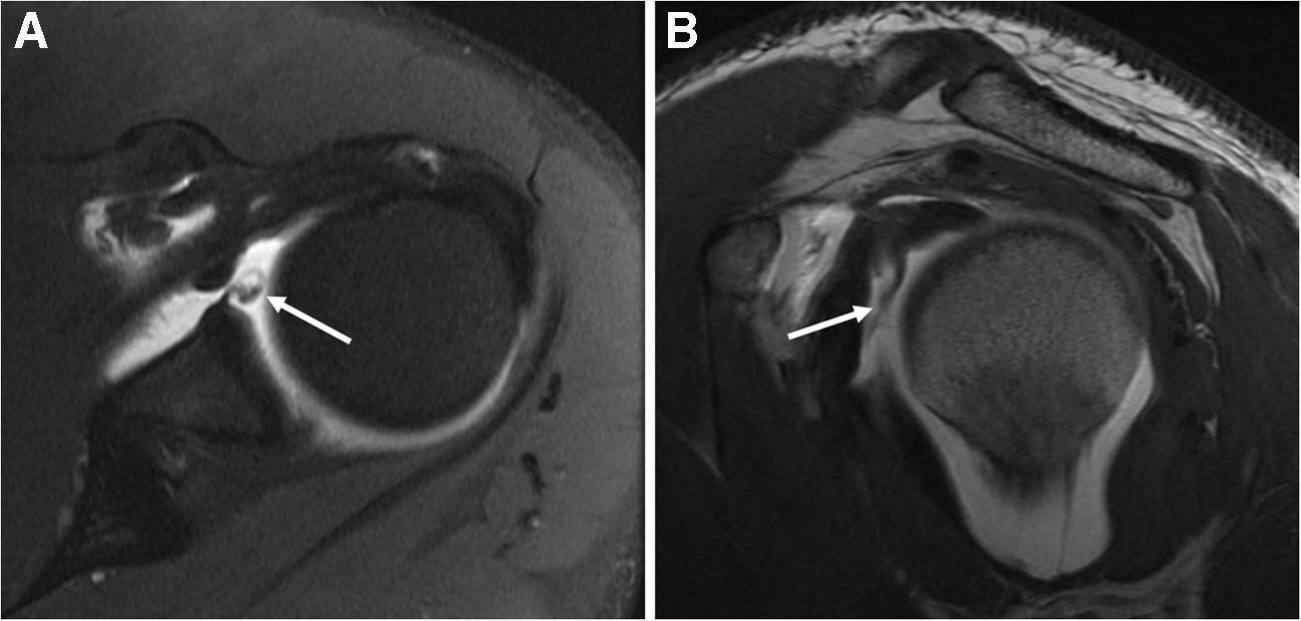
Microinstability (type VI SLAP). Eighteen-year-old man with clinically suspected labral tear. Axial T1-weighted fat-suppressed (A) and sagittal T1-weighted (B) MR arthrogram images show a tear of the anterosuperior labrum with a fragment displaced into the anterosuperior joint recess (arrows)
